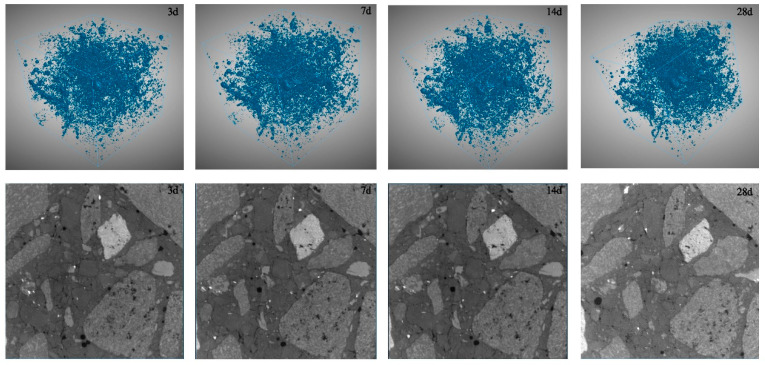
X-CT Image of SCC with 5% CAI dosage.
